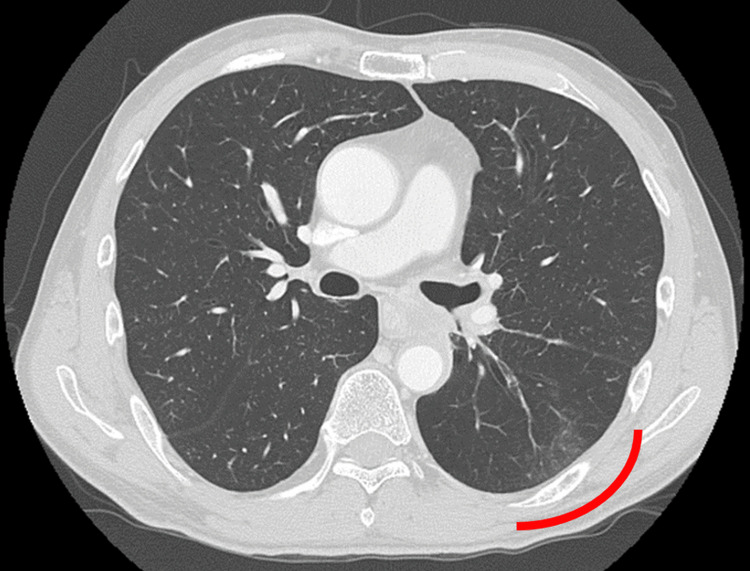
CT image on the same day RP was suggested by the CBCT images, where GGO was confirmed at the left lung S6, as shown with the red line RP - radiation pneumonitis; CBCT - cone-beam computed tomography; GGO - ground glass opacity
